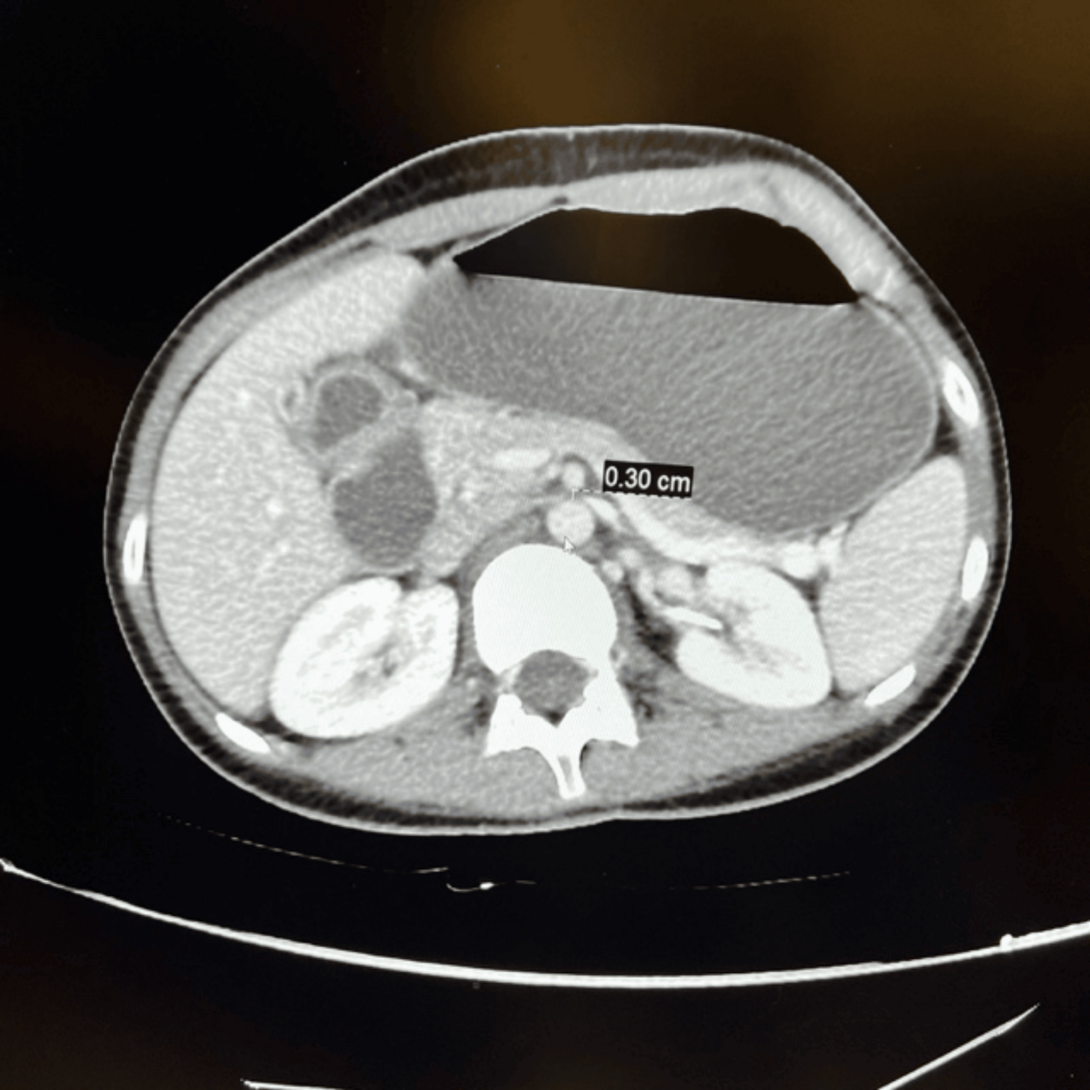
CT axial section showing aortomesenteric distance of 30 mm. CT: computed tomography.
